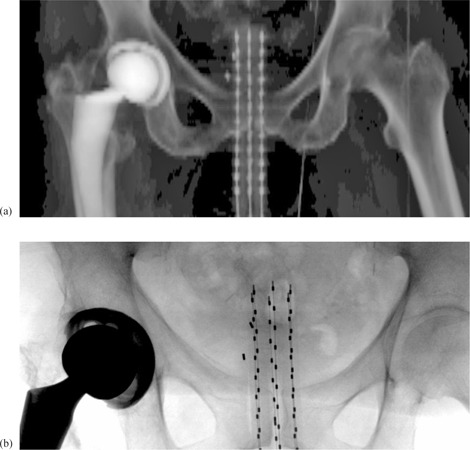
Example of an AP CT scout image (a) used as a baseline; example of an AP digital radiographic image (b) taken before a treatment fraction. Coded dummy wires are placed inside each peripheral channel, as shown.
